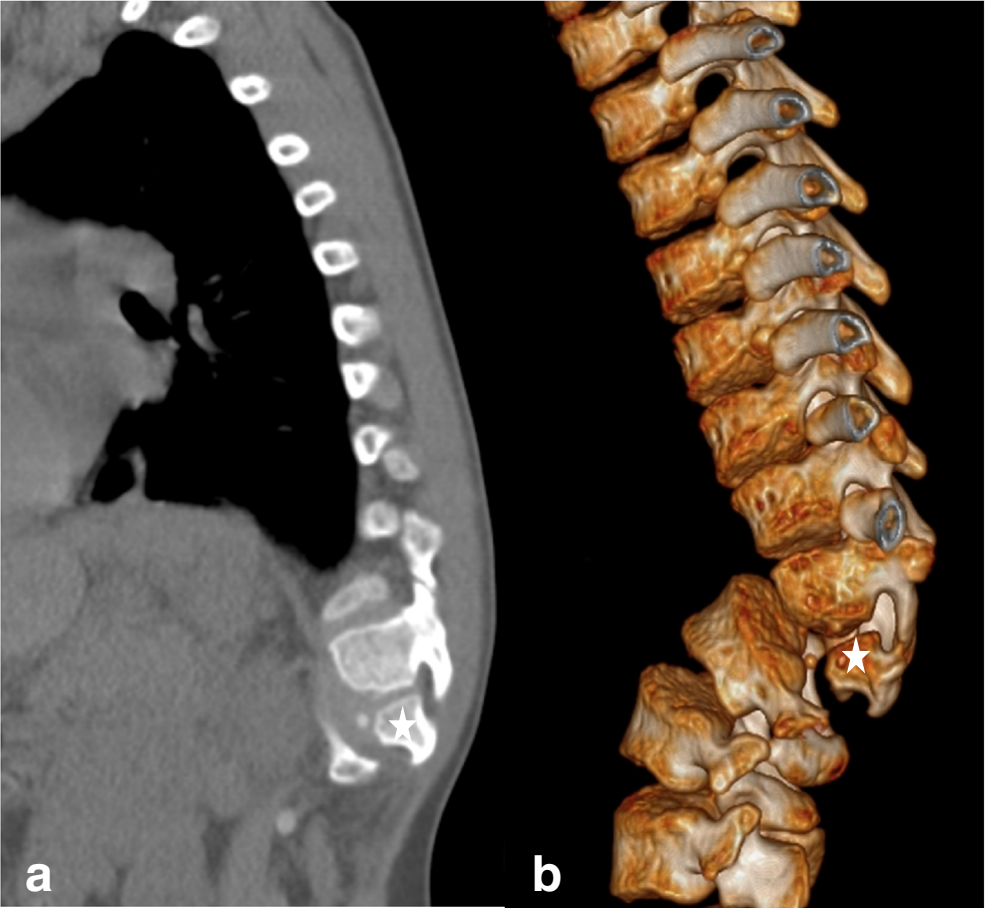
a-b: Wedge vertebra at T12: Sagittal CT (*a*) and volumetric CT reconstruction of the spine (*b*) demonstrate a small, wedge-shaped T12 (*stars* on *a* and *b*) with associated focal kyphosis; there was associated scoliosis (not depicted)
